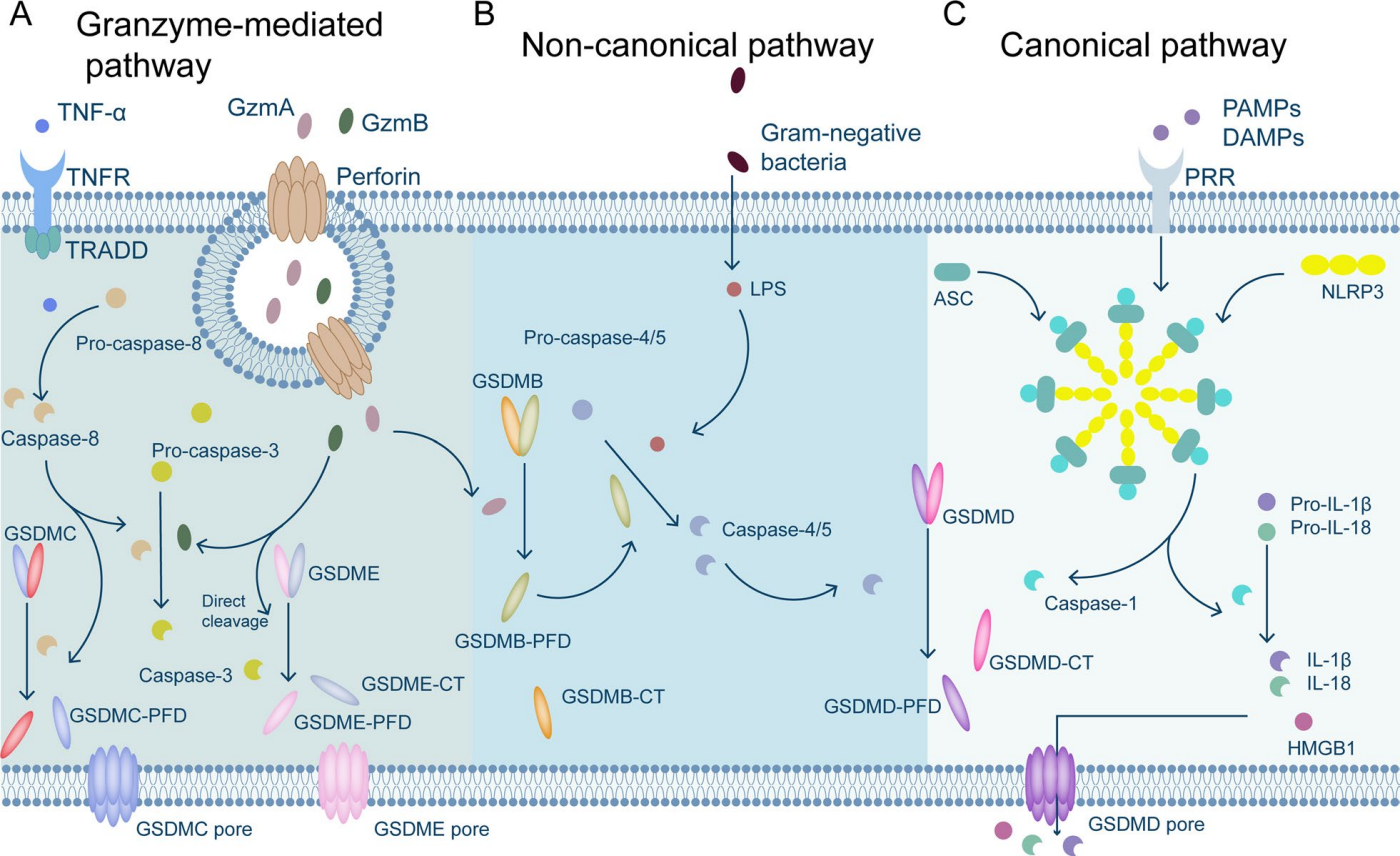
Summary of pyroptosis mediated by different cellular mechanisms. A Pyroptosis induced by the TNF-α/TRADD pathway and the mechanism of pyroptosis induced by granzymes A and B. B Chemotherapy and CAR-T therapy induce cell death mediated by nonclassical pyroptotic pathways. C Interactions of PAMPs and DAMPs with pattern recognition receptors on the cell surface trigger the classical pyroptotic pathway, leading to the release of HMGB1, IL-1β, and IL-18. (Gzm A: Granzyme A; Gzm B: Granzyme B; PRRs: pattern recognition receptors; PAMPs: pathogen-associated molecular patterns; DAMPs: damage-associated molecular patterns; GSDMD-CT: GSDMD-C-terminus; and GSDME-CT: GSDME-C-terminus.)
